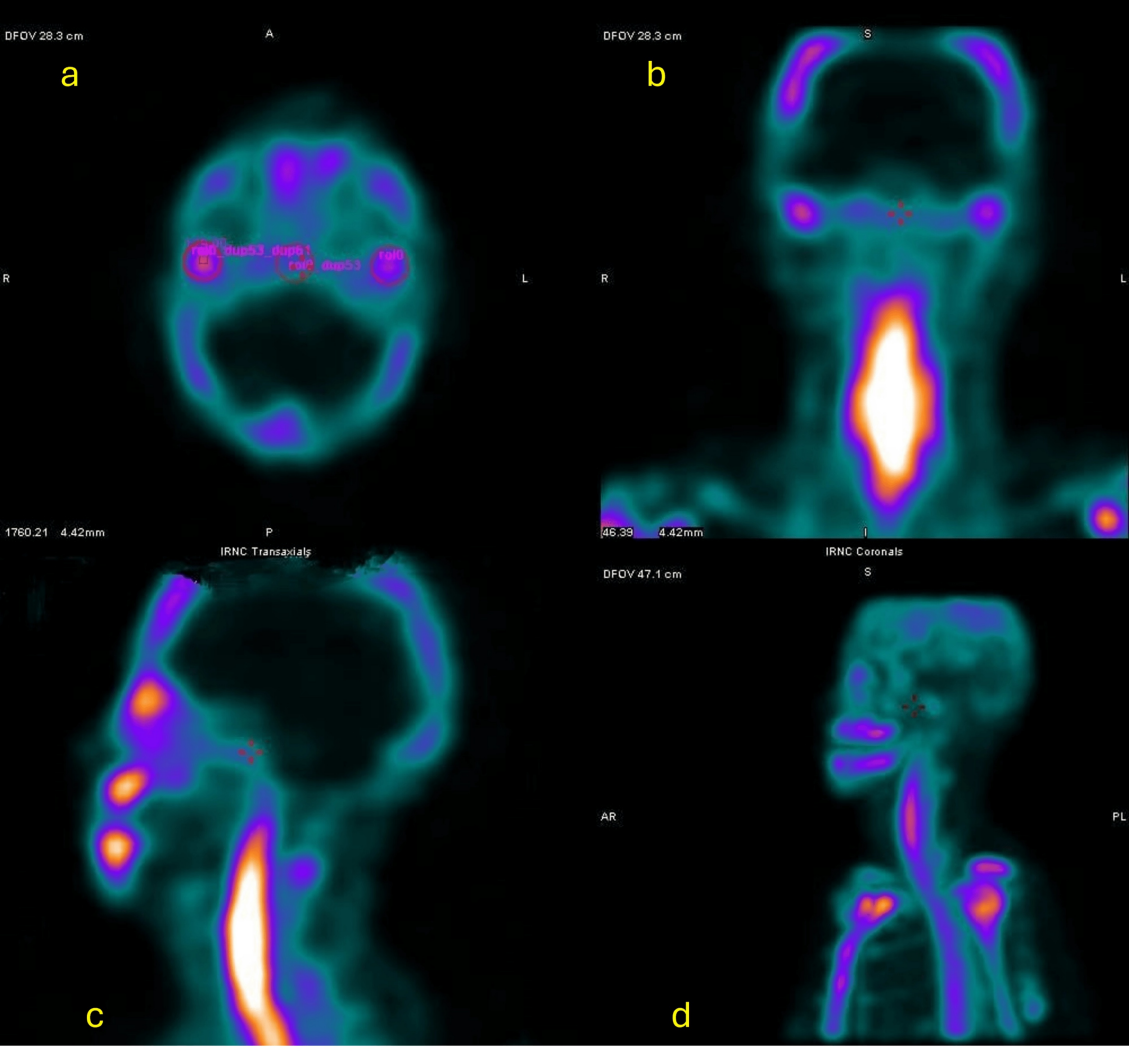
Single photon emission computed tomography (SPECT) imaging of the craniofacial region for functional evaluation of the temporomandibular joints (TMJs). (a) Axial view showing bilateral uptake in the TMJs, indicating active bone remodeling or inflammation.
